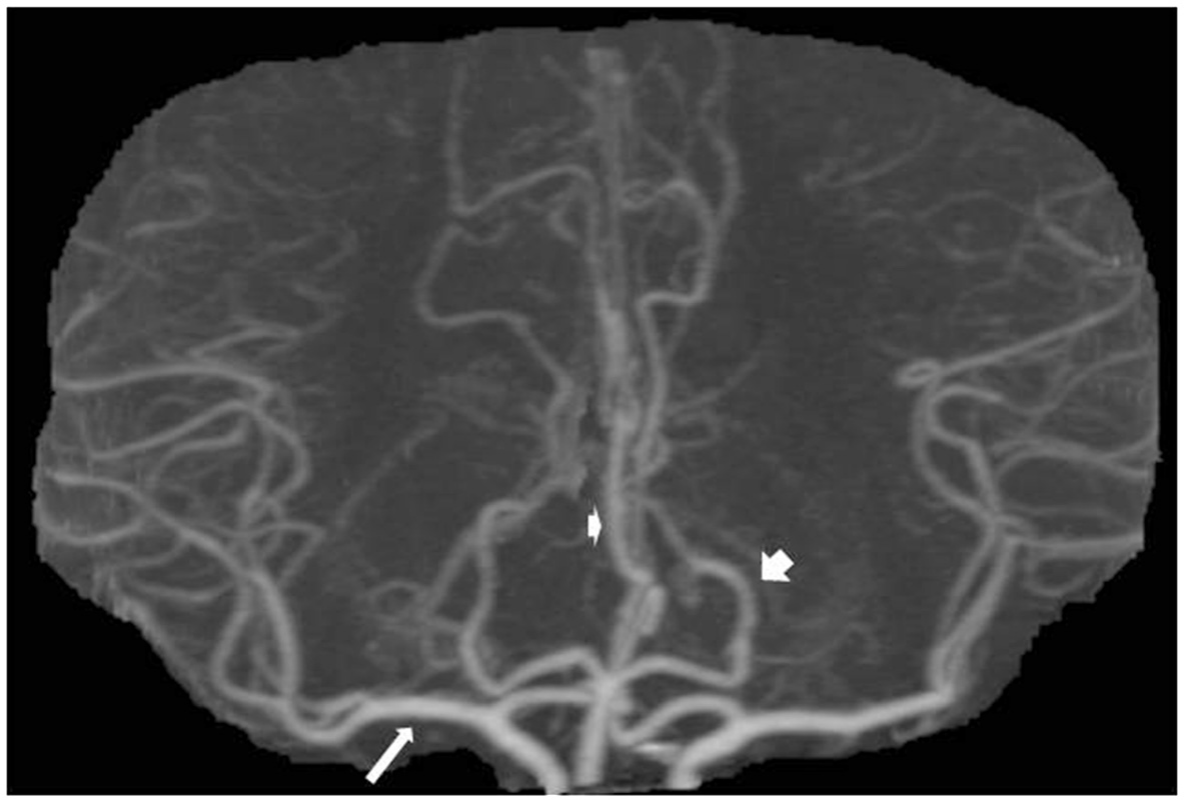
Maximal intensity projection reconstruction of contrast-enhanced computed tomography angiography (frontal view) showing normal vessel: middle cerebral artery (arrow); anterior cerebral artery (smaller arrowhead); posterior cerebral artery (larger arrowhead). The resolution was too low to demonstrate the vessels supplying the thalamus.
